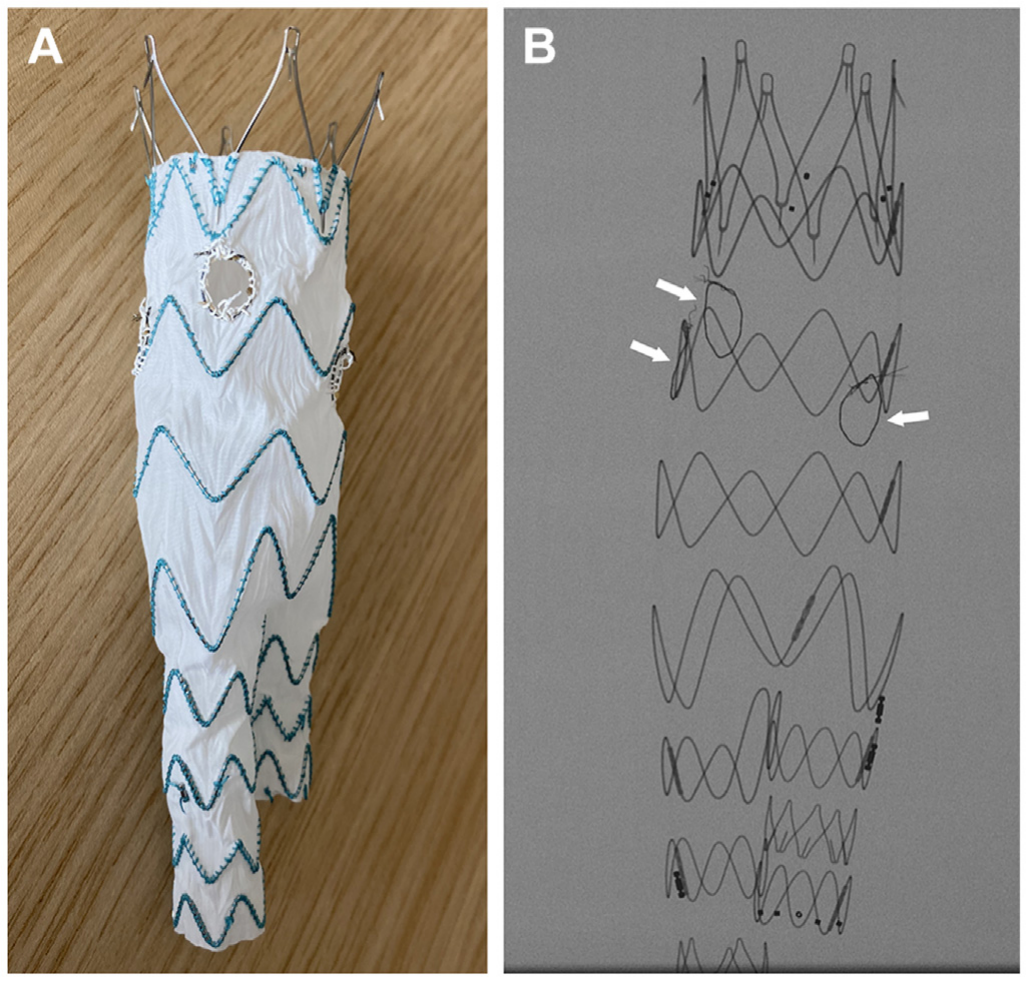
Terumo Aortic TREO device. The wide amplitude stent design and increased interstent distances help physician modification as demonstrated unsheathed on a table (A) and radiographically (B) in a test device implementing three fenestrations.
